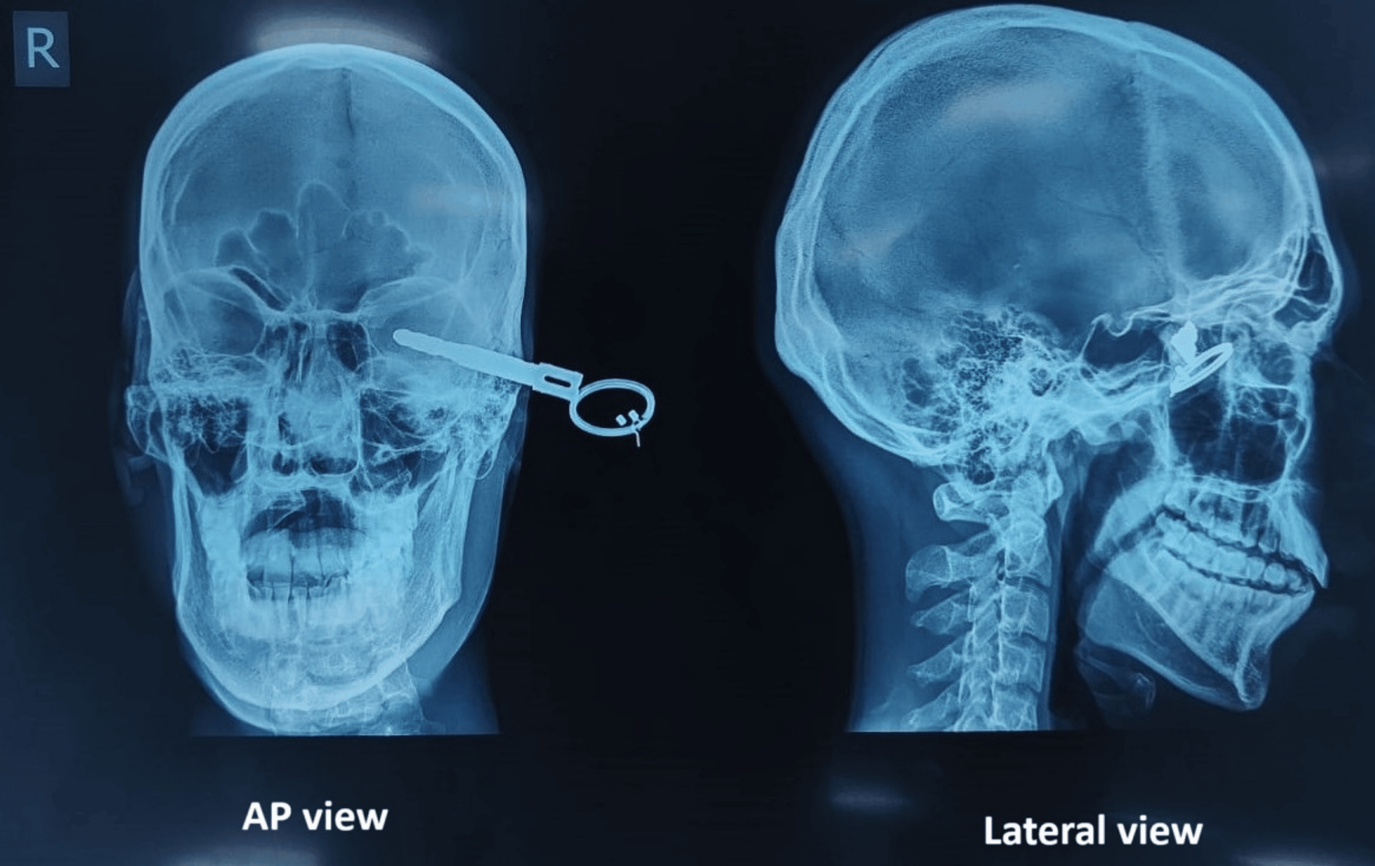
Plain X-ray AP view and lateral view showing the bike key in left orbit.
